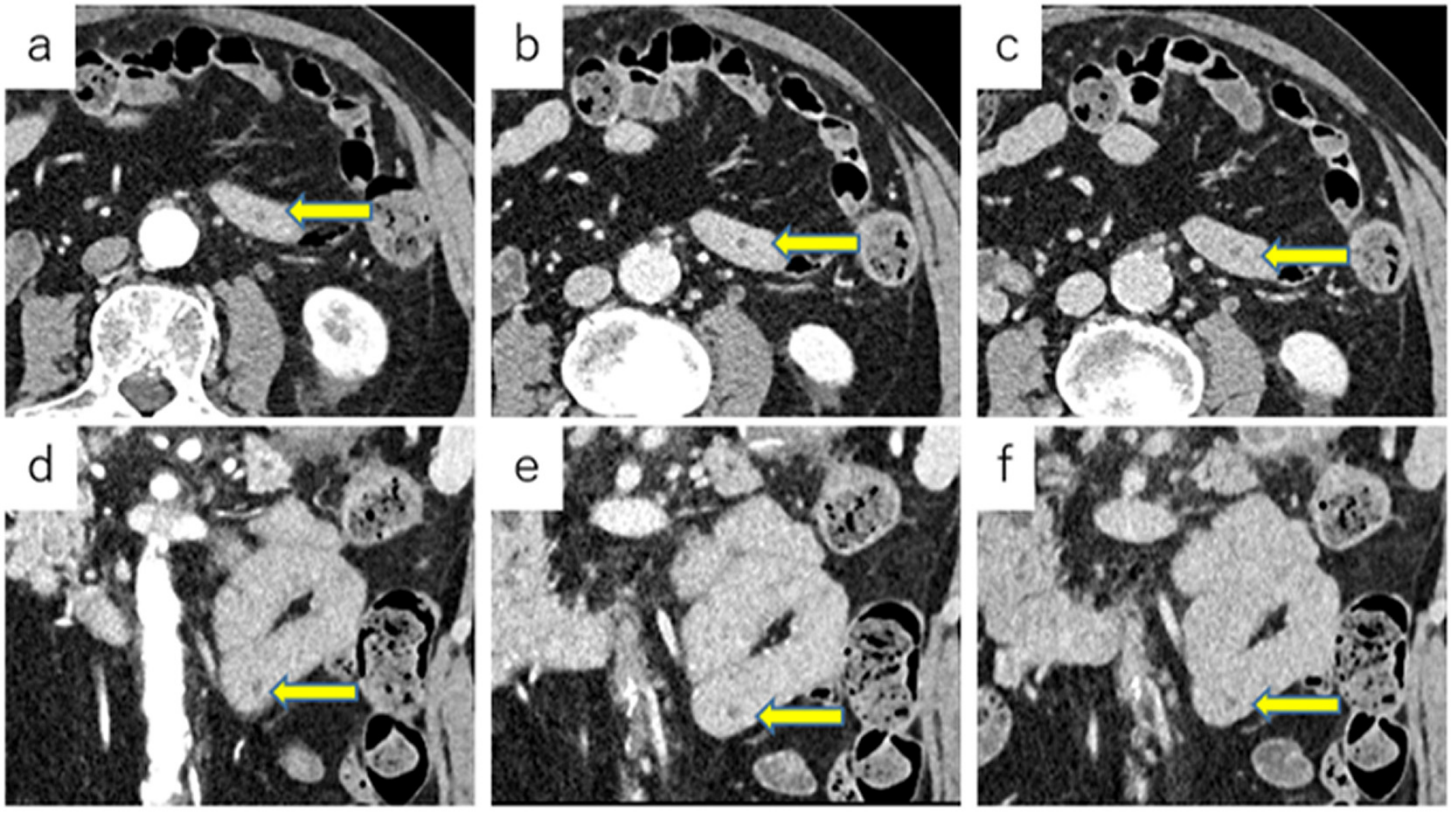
Contrast-enhanced CT scan revealed a homogeneous cystic lesion that was 5 mm in diameter without contrast (arrow). A, Axial view, arterial phase. B, Coronal view, arterial phase. C, Axial view, portal phase. D, Coronal view, portal phase. E, Axial view, equilibrium phase. F, Coronal view, equilibrium phase.
